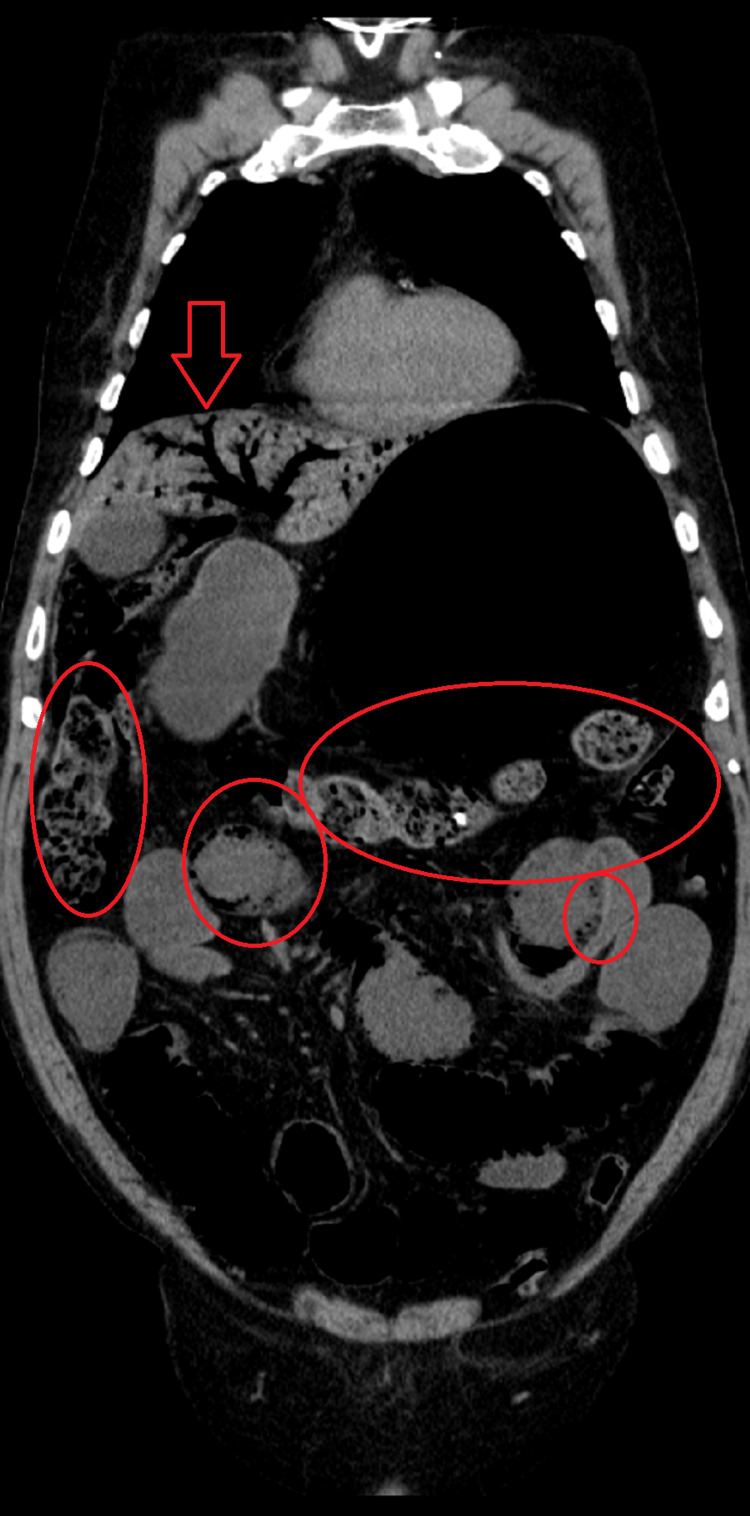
CT scan of the abdomen showing aeroportia (arrow) and intestinal pneumatosis (circle)
